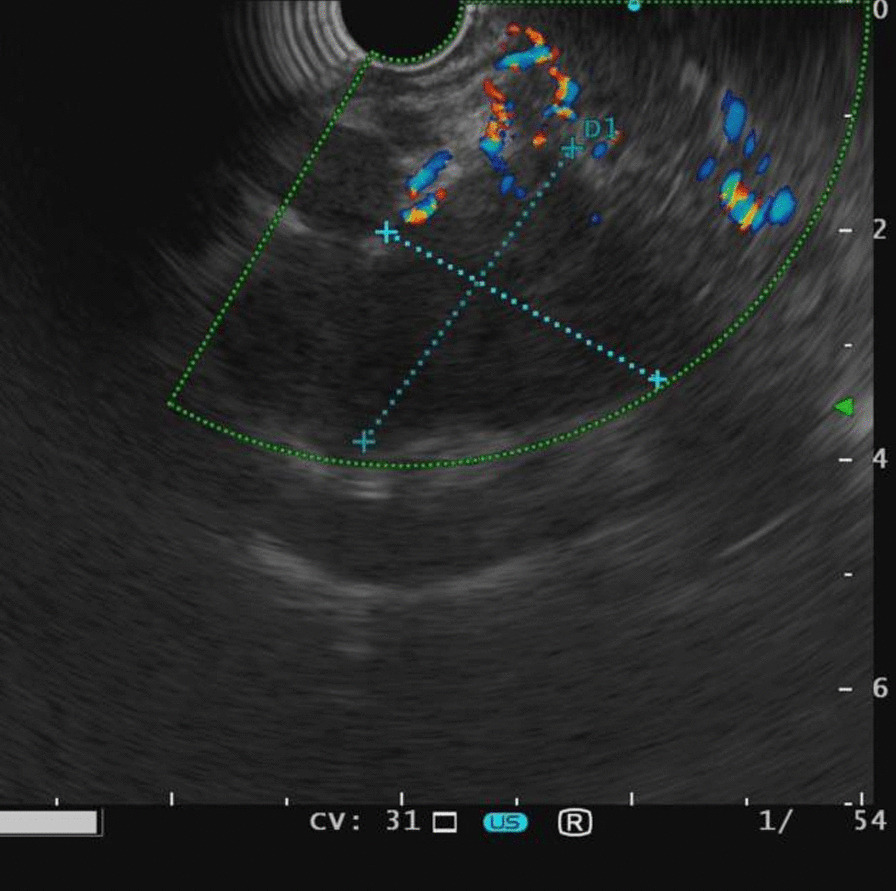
Endoscopic ultrasonography demonstrated an irregular hypoechoic area within the head of the pancreas above, involving the lower common bile duct
